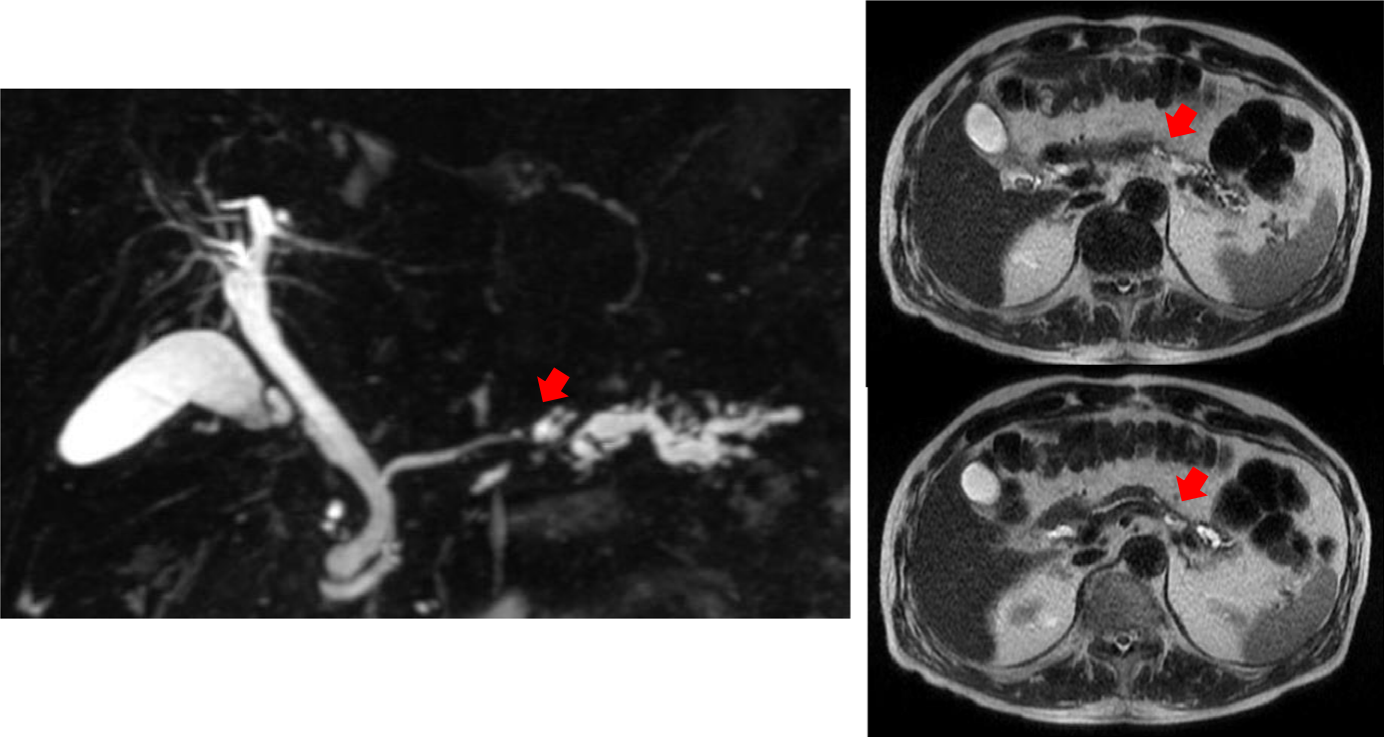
Magnetic resonance imaging. The main pancreatic duct in the body and tail of the pancreas is dilated, but there is no visible tumor
